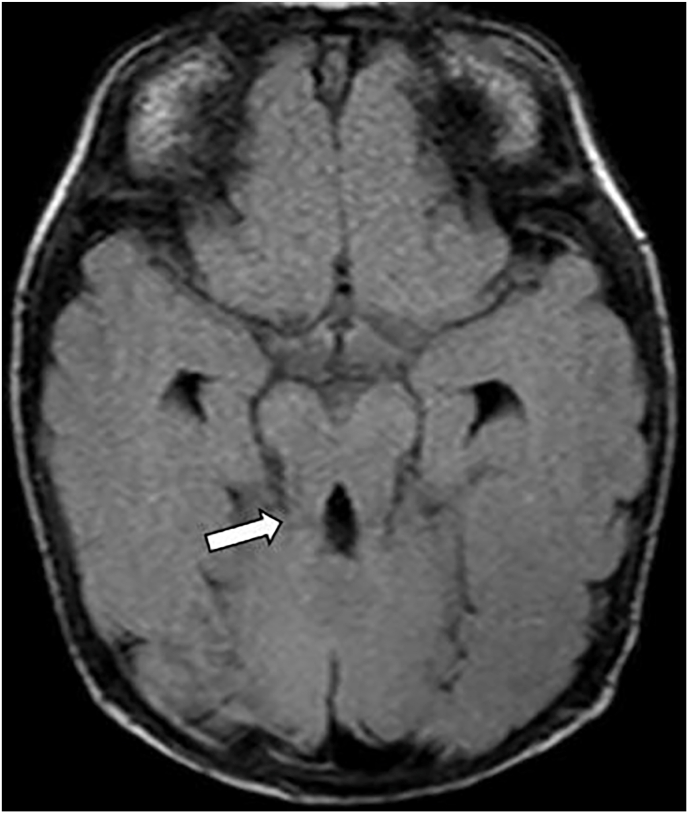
Axial T1 weighted magnetic resonance image (MRI) shows the classic “molar tooth sign” of the midbrain-hindbrain (white arrow) pathognomonic for Joubert syndrome.
